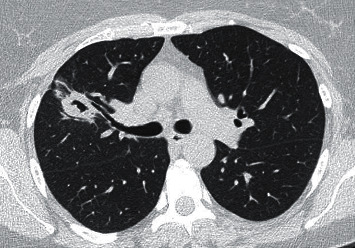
CT thorax (lung window) axial view showing the cavitary lesion in the right upper lobe of the lung.
